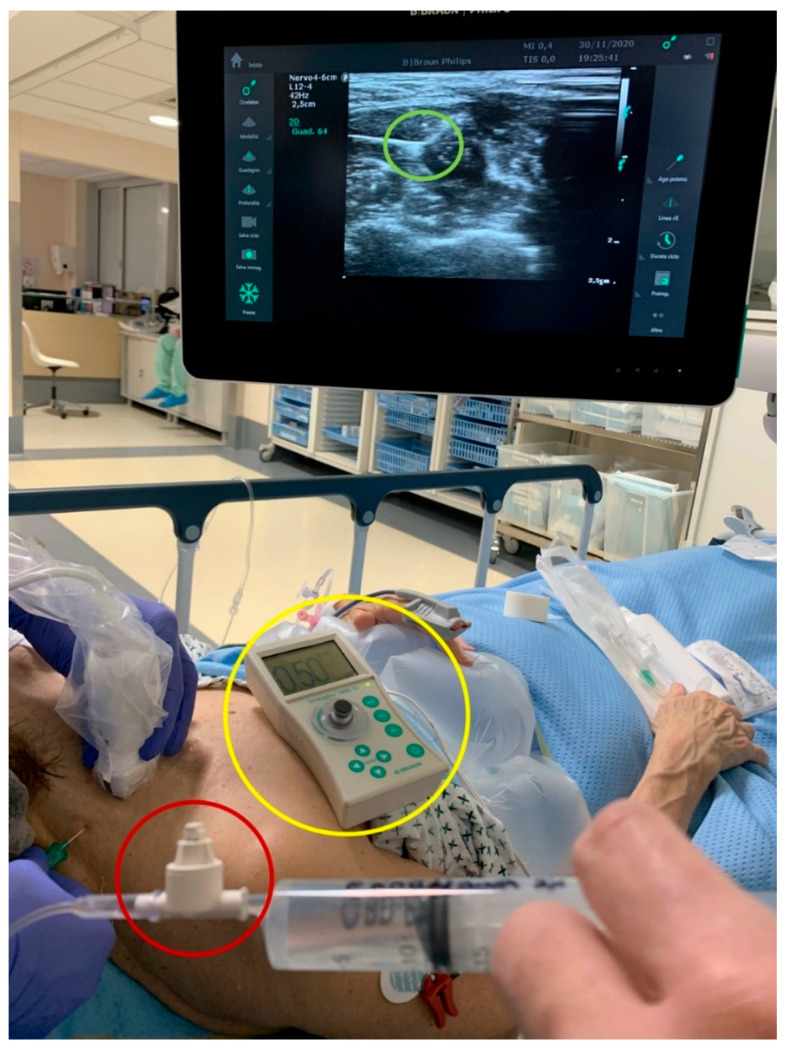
The triple monitoring devices. Green circle: ultrasound guidance; yellow circle: nerve stimulator; red circle: opening injection pressure monitoring device (BSmart^®^).
